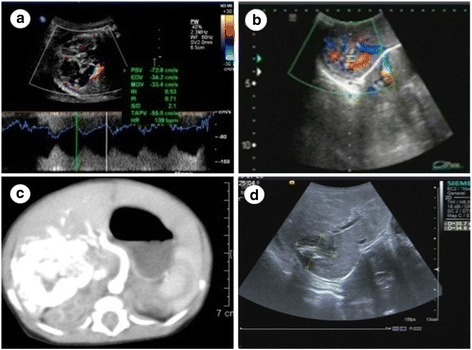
Fetus with a hemangioma in the right liver lobe (fetus 4). a Hypoechoic lesion measuring 56 × 42 × 41 mm. Color Doppler ultrasound shows a strip of blood flow at the edge of the lesion and stars intralesion. Pulse Doppler ultrasound suggests that the resistance index is 0.53. b Ultrasound on the day of birth shows that the lesion is less clear, with nonuniform density and punctate calcifications. The hepatic vein was bypassed. c Postnatal computed tomography (CT) shows nonuniform density and punctate calcifications. On contrast-enhanced CT, the lesion was centripetal enhancement. It was supplied by a branch of the hepatic artery and drained via the hepatic veins; the right and middle hepatic veins were thickened. d Six months after treatment of the neonate with propranolol and dexamethasone, the lesion was 28 × 25 × 27 mm in size. Calcification plaques were observed, and the intrahepatic and the extrahepatic bile ducts were not dilated
